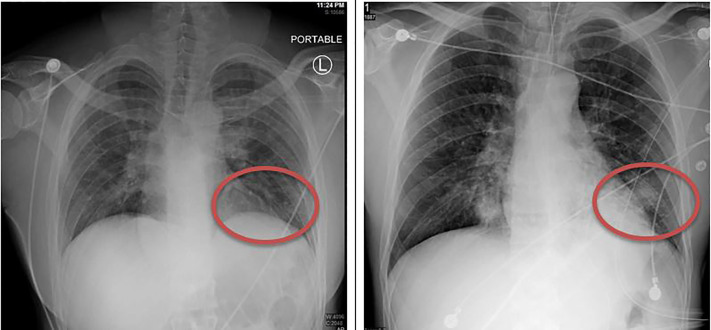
Initial chest radiograph (left) and post-intubation chest radiograph (right) demonstrating interval progression of bibasilar opacities and interstitial opacities in several hours.
